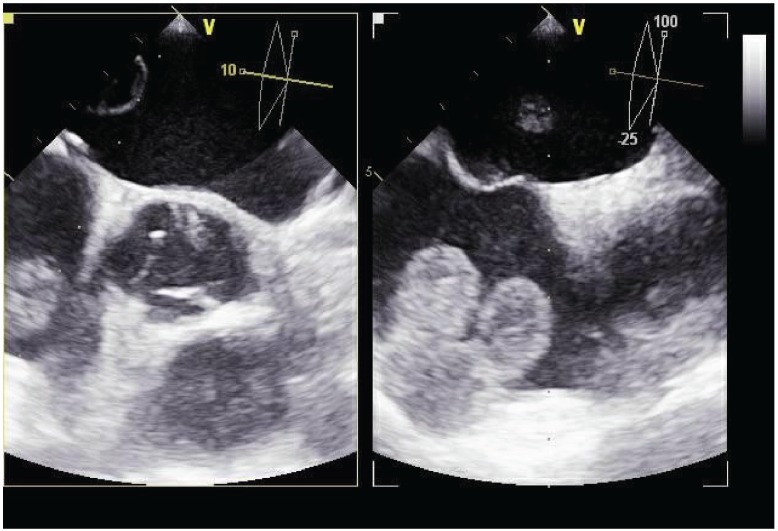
Transesophageal echocardiogram of the mass in the right atrium.
